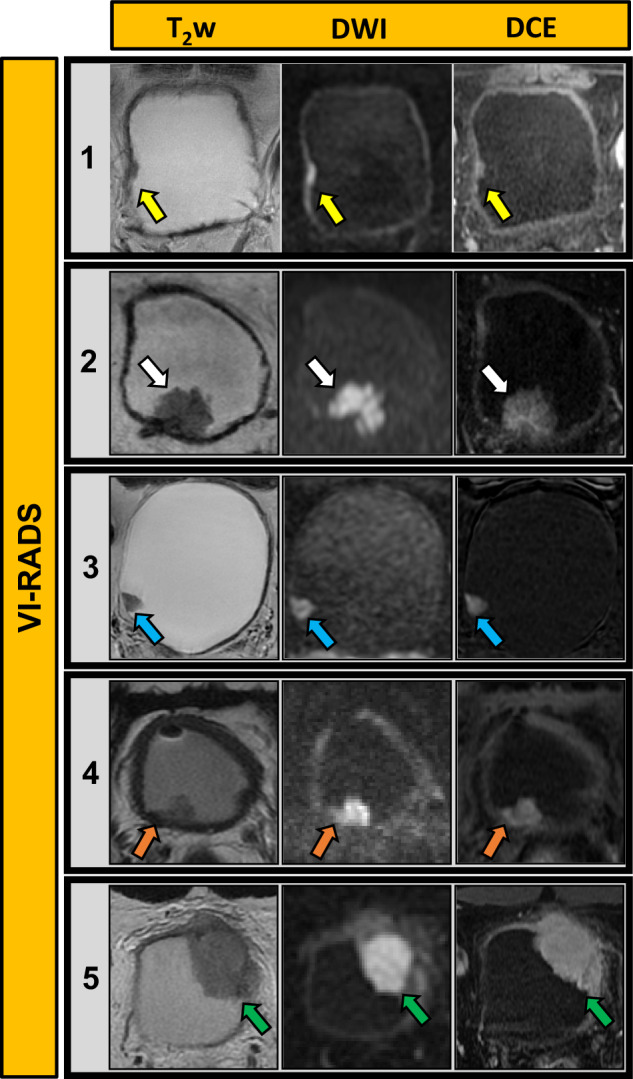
VI-RADS scoring in representative bladder cancer patients. VI-RADS 1: Lesion size < 1 cm; VI-RADS 2: Exophytic tumor with stalk or sessile/broad-based tumor with thickened inner layer; VI-RADS 3: No category 2 findings, but no clear disruption of muscularis propria; VI-RADS 4: Extension of the tumor tissue into the muscularis propria; and VI-RADS 5; Extension of the tumor tissue into the extravesical fat. BCa, bladder cancer; DCE, dynamic contrast-enhanced; DW, diffusion-weighted; T2w, T2-weighted imaging; VI-RADS, Vesical Imaging Reporting and Data System
